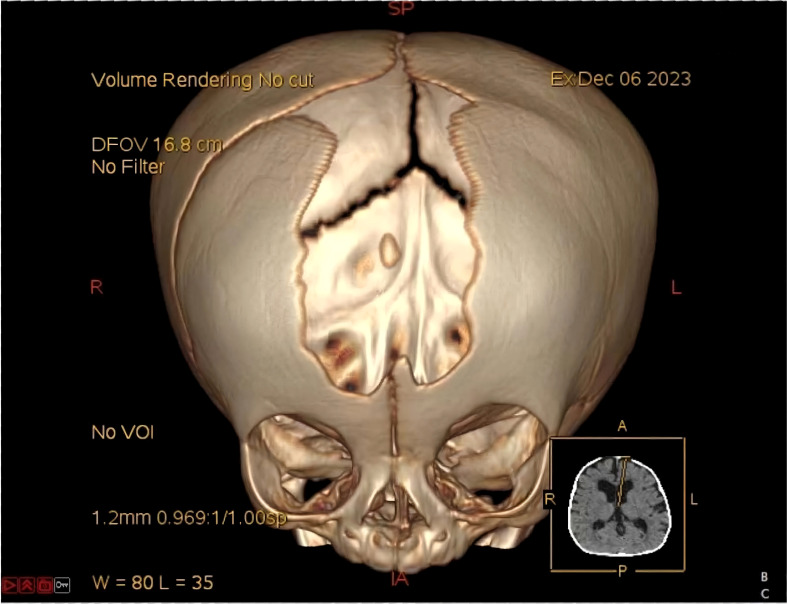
Three-dimensional cranial CT imaging of the patient.
